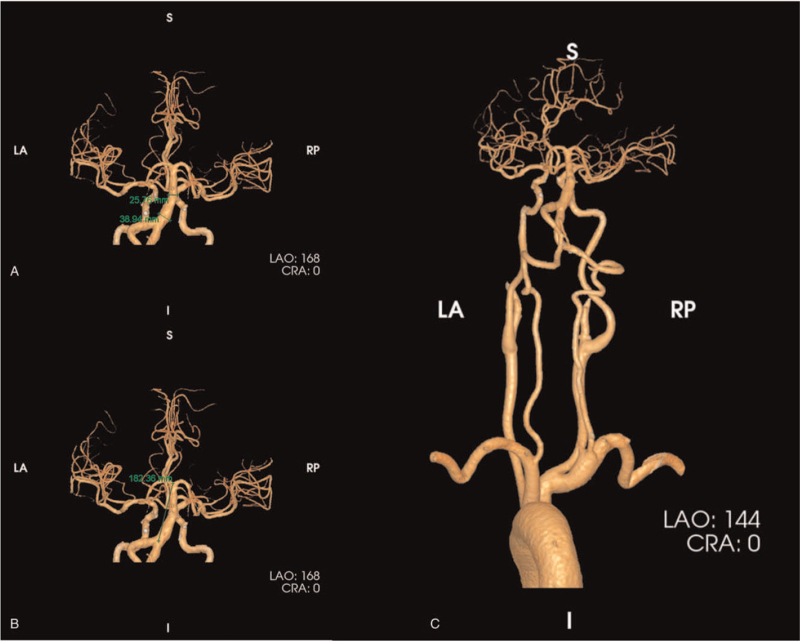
CTA of the intracranial and extracranial arteries. (A) From the 168° LAO perspective, the basilar artery was significantly dilated. Using workstation software, the diameter of the widest portion of the basilar artery was approximately 38.94 mm, and the diameter of the middle portion was approximately 25.76 mm. (B) Due to the tortuosity of the basilar artery, its actual length was greater than 182.36 mm. (C) The remaining intracranial and extracranial arteries were essentially normal. CTA = computed tomography angiography, LA = left anterior, LAO = left anterior oblique view, RP = right posterior.
